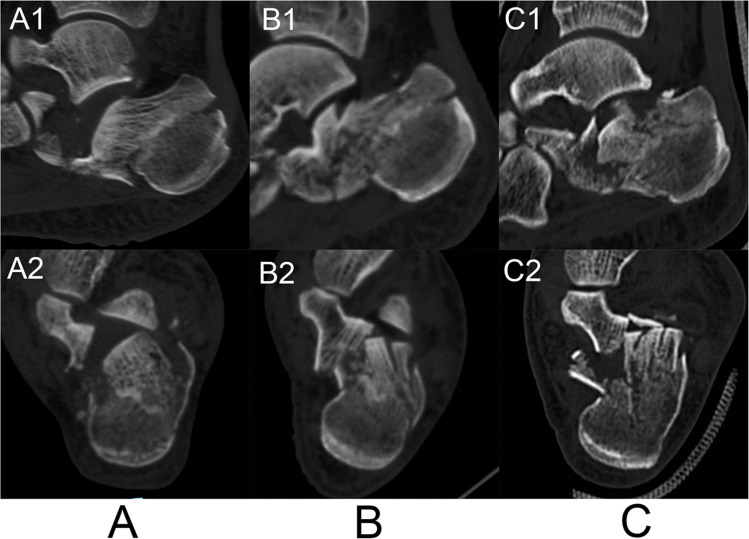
Intact tuberosity fragment in tongue-type fractures. A–C Sanders types II, III, and IV, respectively. (A1, B1, C1) Sagittal CT scans. (A2, B2, C2) Coronal CT scans. CT, computed tomography
